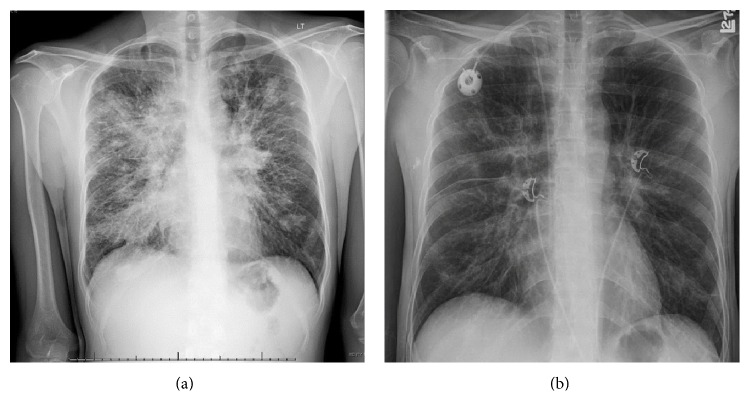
Chest X-ray with evidence of bilateral pulmonary opacities and hilar fullness on presentation (a) and after 4 cycles of VAC/IE chemotherapy (b).
